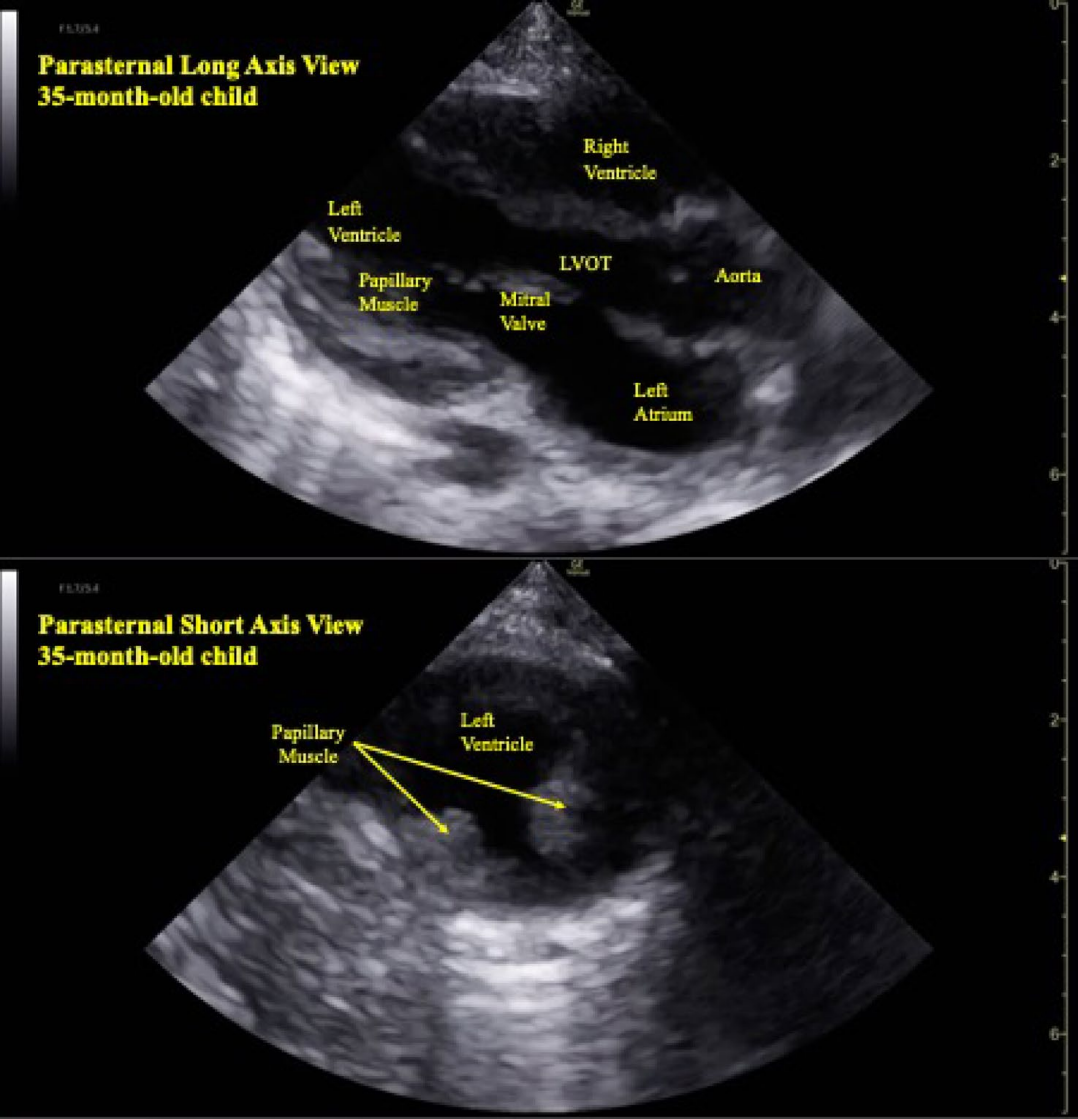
Representative parasternal long-axis and short-axis echocardiographic images for identifying the position of the left ventricle. Parasternal long-axis view and short-axis transthoracic echocardiographic images clearly visualize the left ventricle, left ventricular outflow tract, and papillary muscles, aiding in accurate identification of left ventricular position. LVOT, left ventricular outflow tract.
